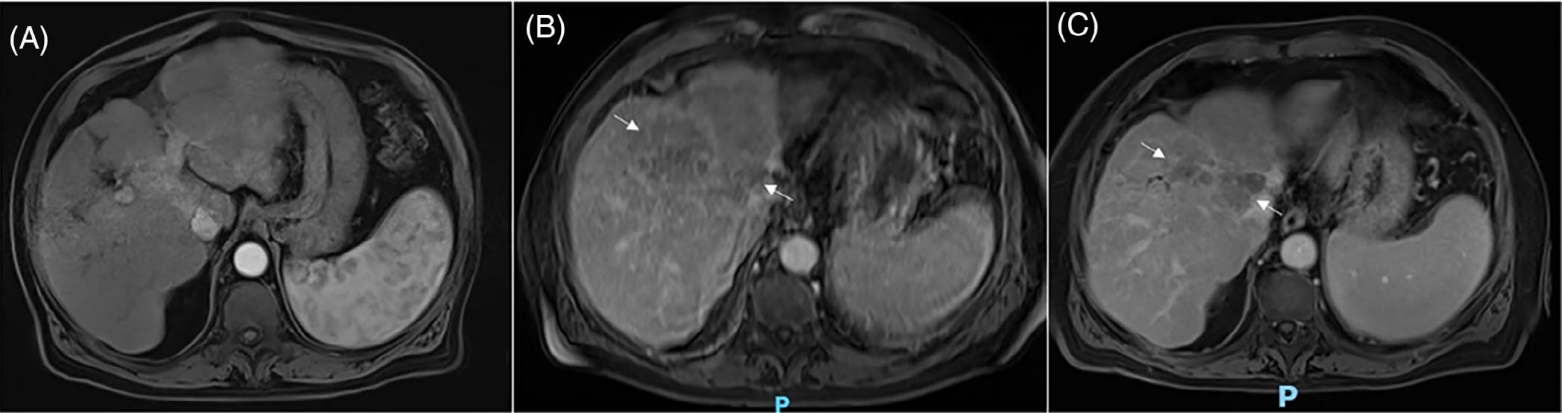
Abdominal MRI. (A) A mass measuring 6.9 × 3.7 cm involving segments 8 and 4A near the IVC with extension into the left and right portal veins can be seen (*arrows*). (B) MRI two months later demonstrated significant tumor growth to 10.0 × 5.8 cm. (C) Follow‐up MRI after four cycles of treatment showed partial tumor response with a reduction to 8.6 × 3.5 cm. There remains a persistent thrombus within the right hepatic veins with some extension into the suprahepatic IVC, overall decreased from prior scans.
